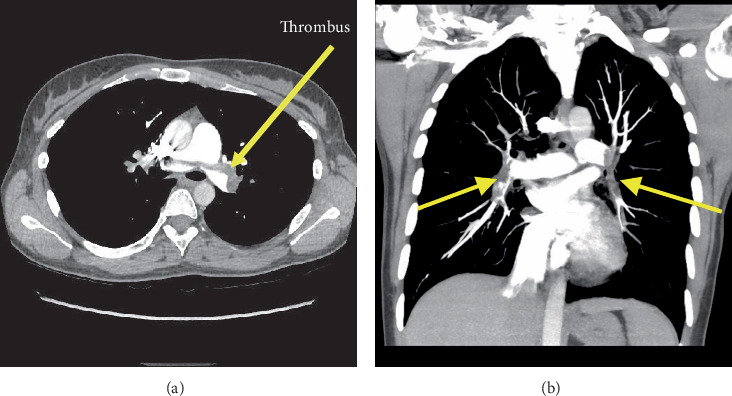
Computed tomography pulmonary angiogram (CTPA). (a) Axial image. Low-density (grey) filling defect noted across the main pulmonary arterial trunk at its bifurcation. (b) Coronal image. Bilateral pulmonary emboli extending into all lobar and segmental branches. Arrows identify thrombus.
